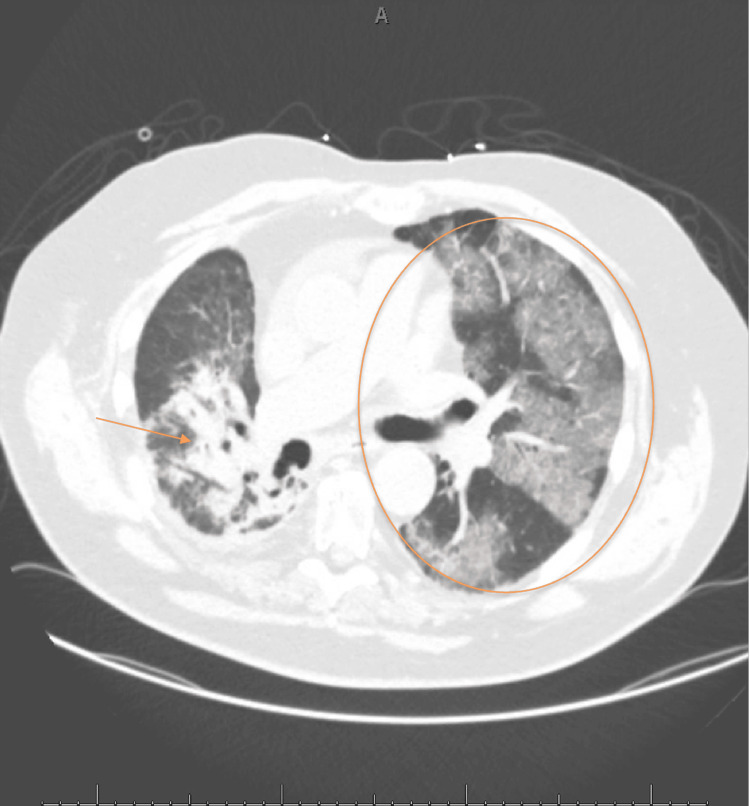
Computed tomography of the chest on admission Axial computed tomography scan of the chest demonstrating bilateral ground-glass opacities and interstitial changes (outlined area) with focal right-sided consolidation (arrow), described based on radiographic appearance as consistent with a nonspecific interstitial pneumonia pattern. Image interpretation was performed as part of routine clinical radiology evaluation without use of proprietary imaging analysis software or commercial classification tools.
